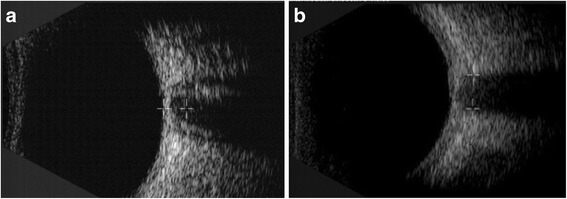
The scan images of ONSD measurement with B-scan ultrasound. a is the picture of ultrasonic measurement of ONSD in a high intracranial pressure patient. In this picture, there is a more significant subarachnoid space around optic nerve. b is the picture of ultrasonic measurement of ONSD in a normal intracranial pressure patient
